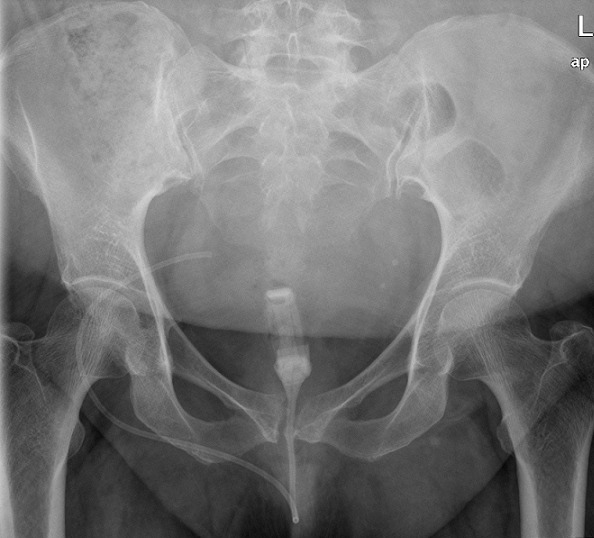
Pelvic X-RAY hours after the CT showed the gastric band and its connecting tube in the lower pelvis
